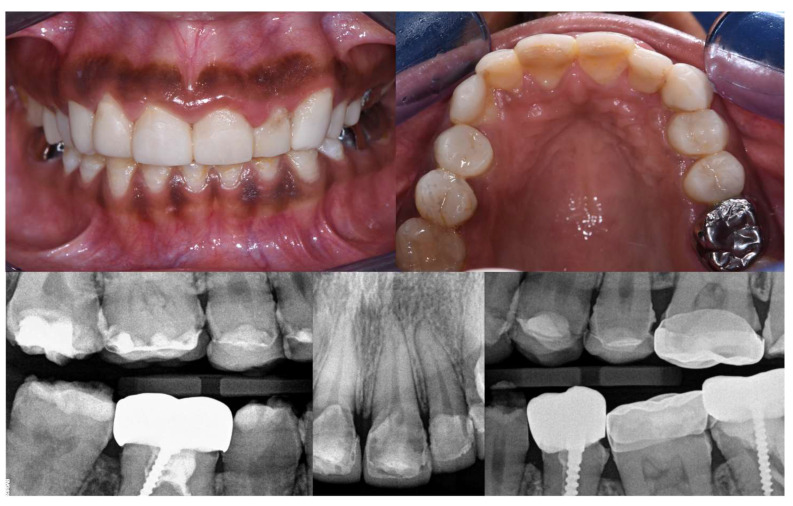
Intraoral views and radiographs at 8-month follow-up.
